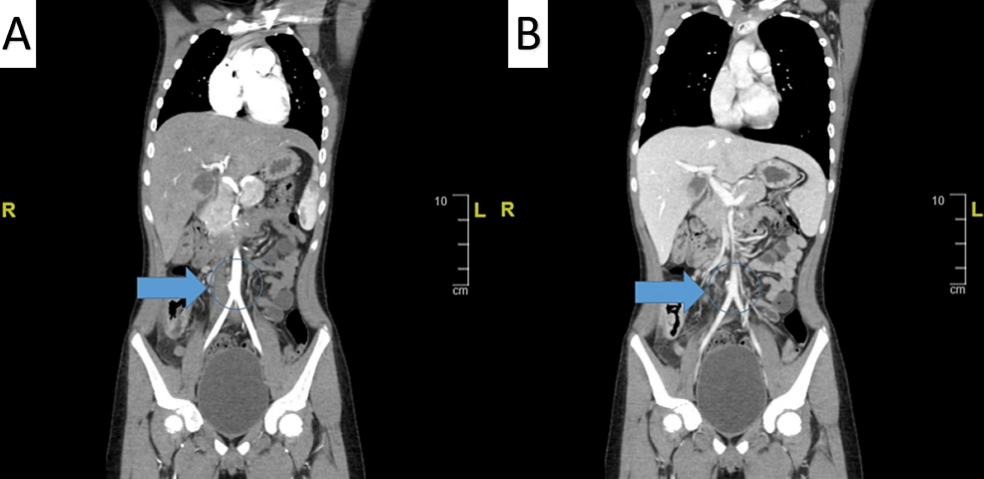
Postoperative 4-Years Follow-Up Computed Tomography Contrast Scans A: Demonstrating no residual findings (blue arrow); B: No evidence of contrast extravasation (blue arrow).
